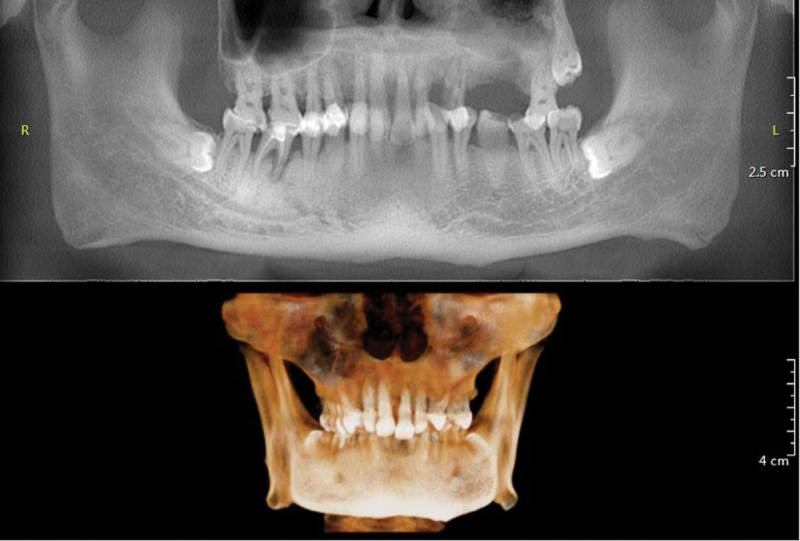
CBCT revealed that the full mouth alveolar bone was absorbed to varying degrees into the middle 1/3 of the root.
